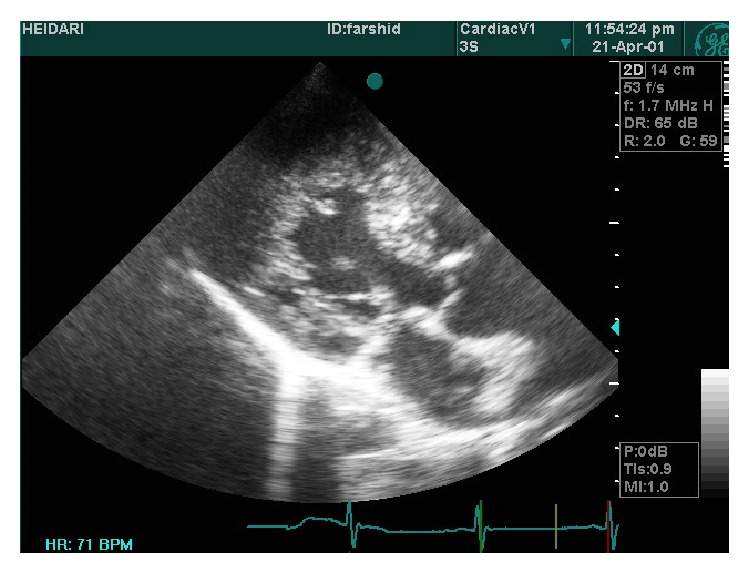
Parasternal long axis view showed hypertrabeculation, antroseptal and posterior wall.
